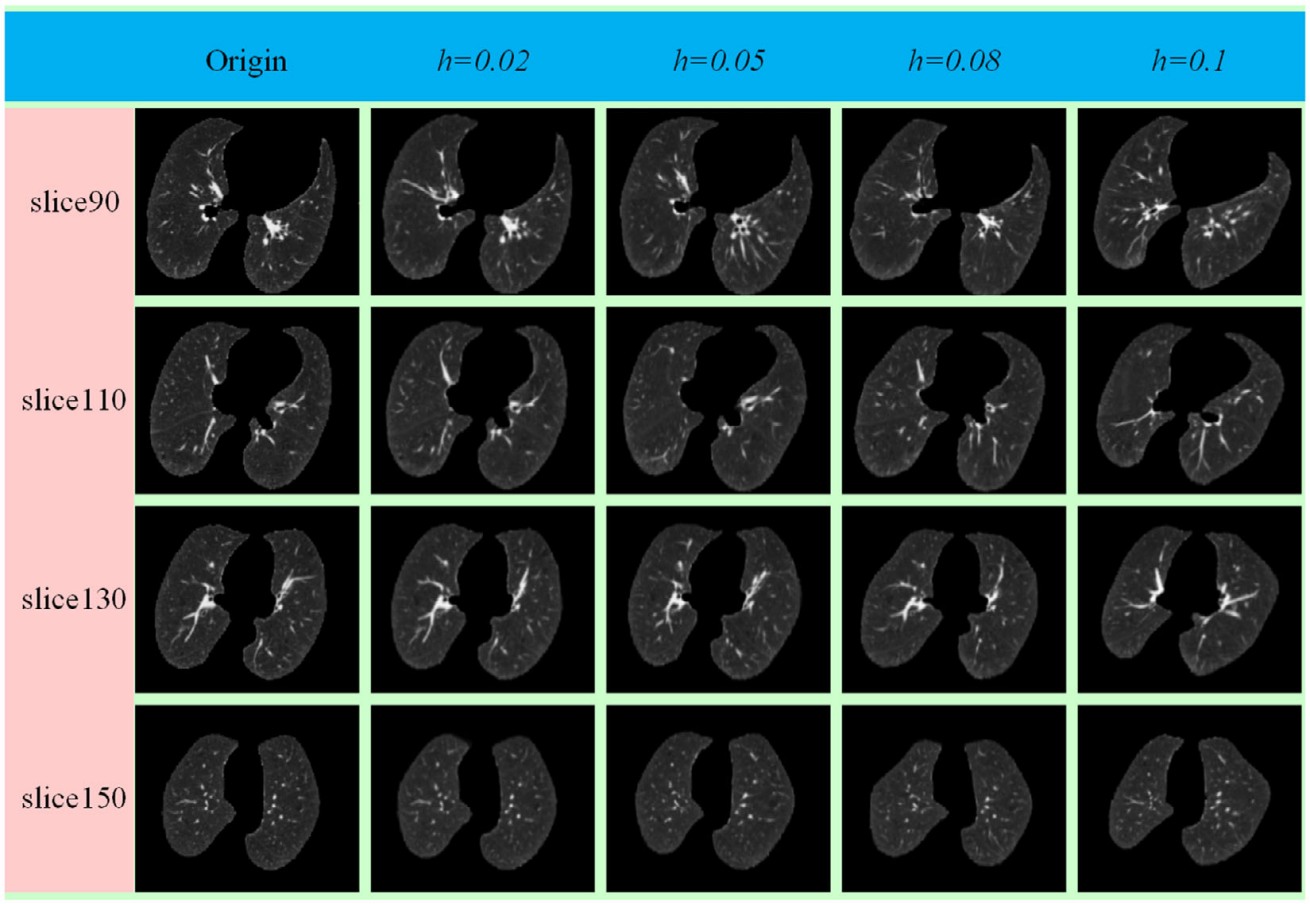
Examples of augmented data of 3D chest CT images from the EMPIRE10 dataset. From top to bottom, the different slices of the axial plane are shown. From left to right, the original images and the transformed images with different scales are shown
